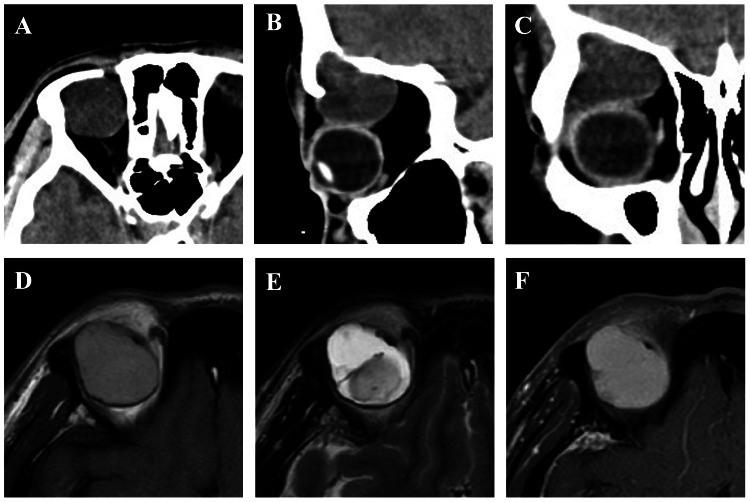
CT and MRI findings (A–C) Computed tomography (CT) scans: (A) axial, (B) sagittal, and (C) coronal sections showing a well-circumscribed 3.0-cm mass in the superolateral right orbit; (D–F) Magnetic resonance imaging (MRI): (D) T1-weighted, (E) T2-weighted, and (F) gadolinium-enhanced T1-weighted images demonstrating a hyperintense mass on both T1- and T2-weighted sequences, with minimal contrast enhancement. The lesion exhibits internal heterogeneity.
